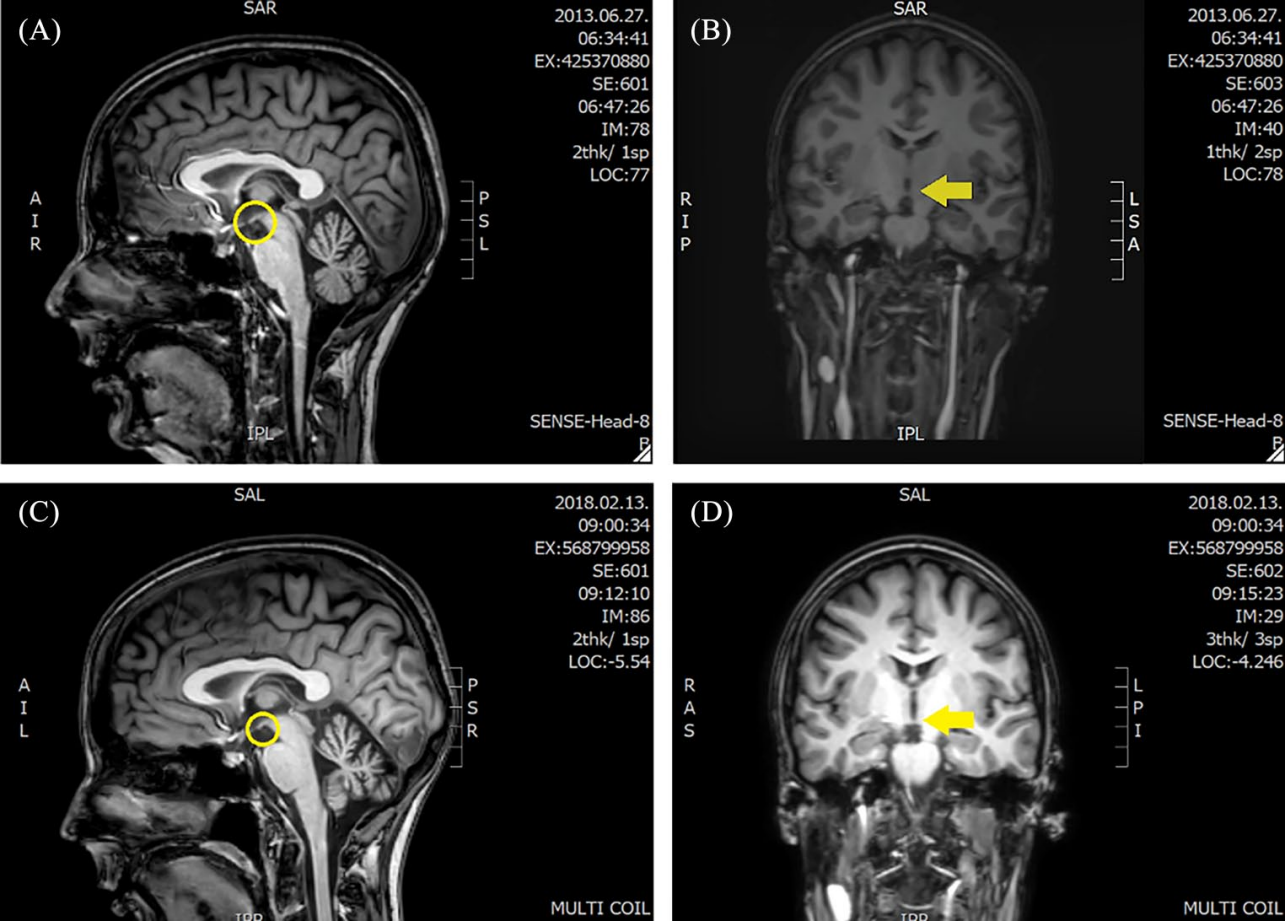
The follow up of the brain MRI. (A, B) An MRI scan was performed in 2013 on a 1.5 T MR, almost absent mamillary bodies, and widened cerebellar sulci are visible. On (C, D), which were performed in 2018 on a 3 T MR, slightly more widened cerebellar sulci and the same atrophic mammillary. MRI, magnetic resonance imaging.
